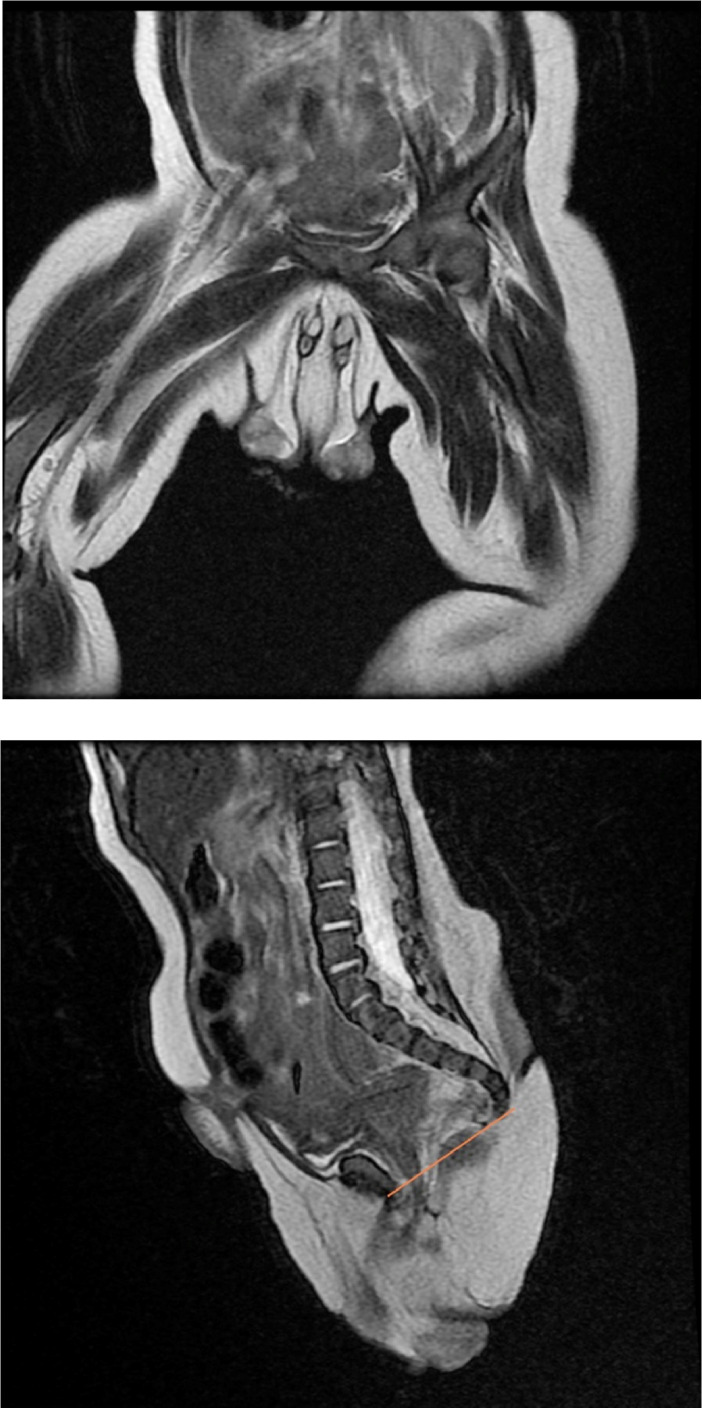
Coronal and sagittal T2-weighted MR image show 2 testes with a well-visualized structure of each penis and the position of the distal rectal pouch compared to the puborectalis muscle.
